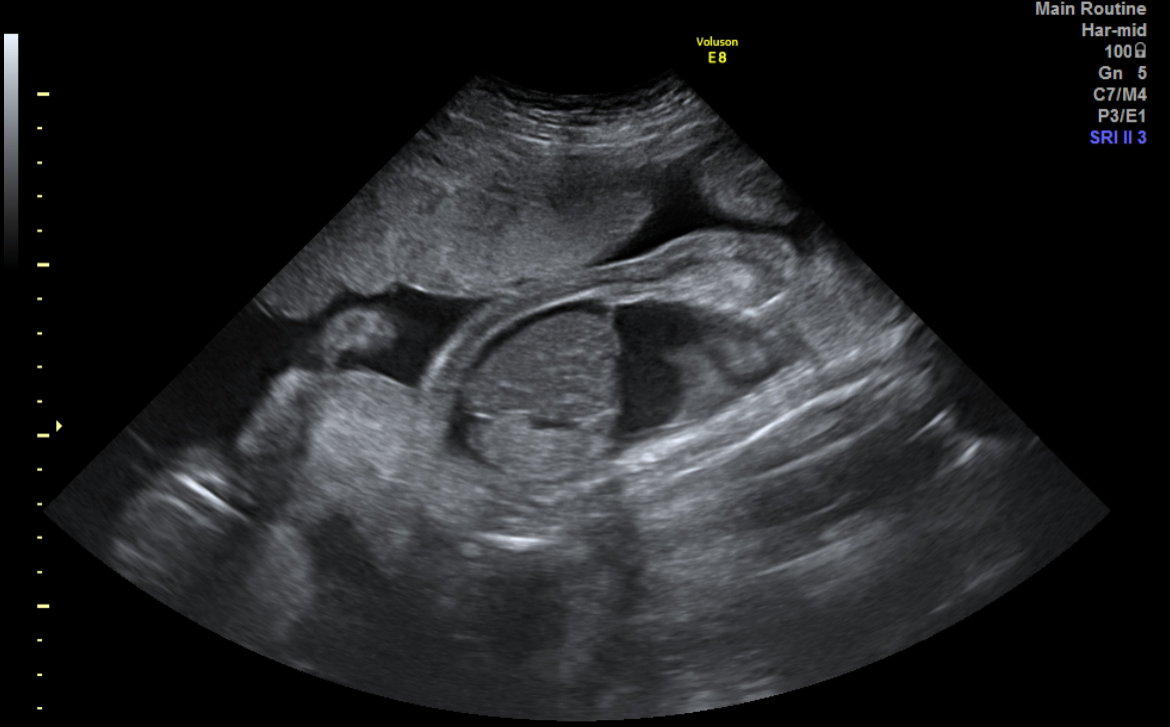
Ultrasound examinations on day 21 after FLP showed severe hydrops fetalis with skin edema, pericardial effusion, and ascites in fetus A (ex‐donor). Edematous placenta was also noted.
